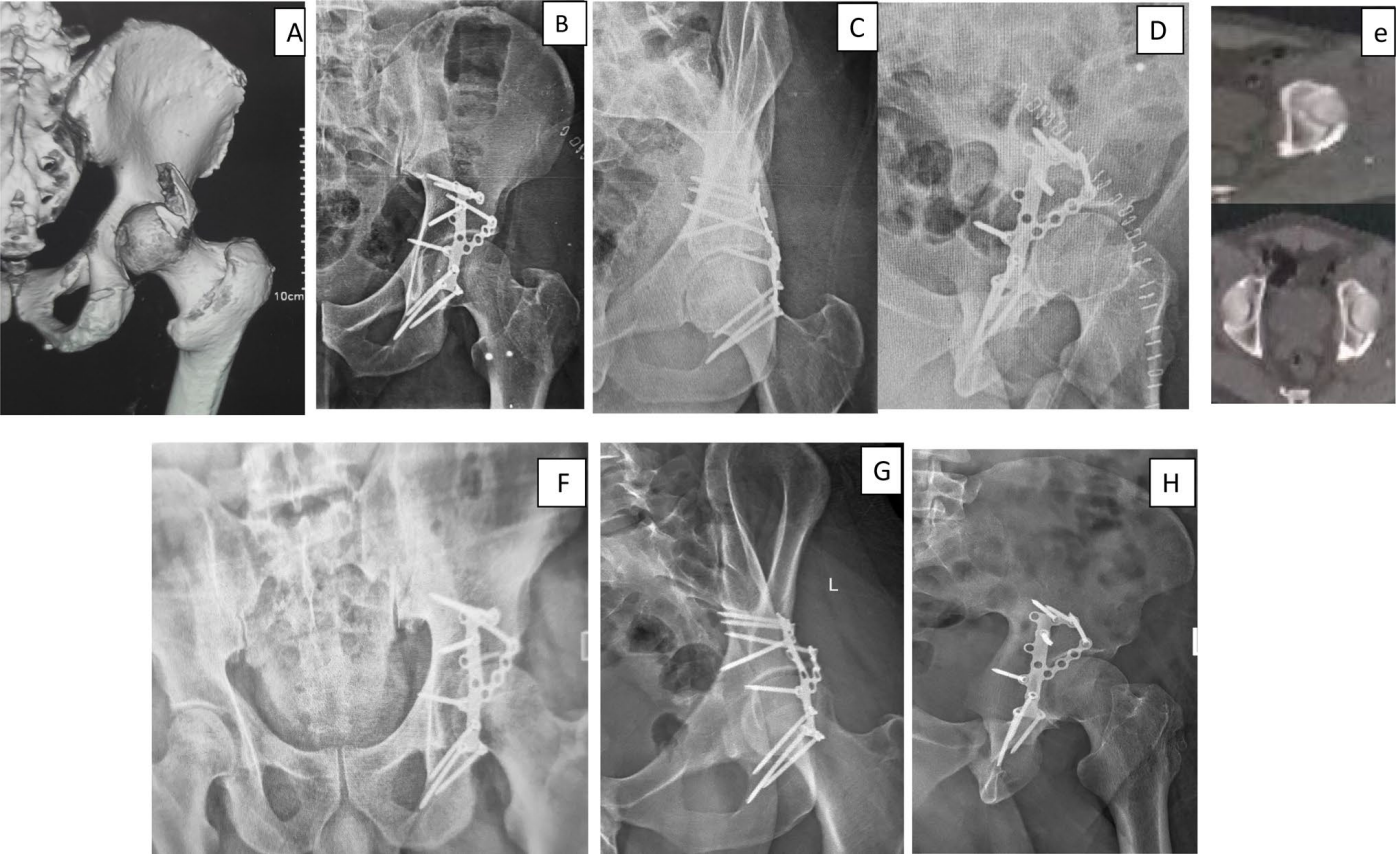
A 3D CT scan of 41 year-old male patient showing comminuted PW fracture of the left hip. B, C, D Postoperative radiograph (AP and Judet views) after calcaneal plate fixation showing anatomically reduced fracture. E Postoperative CT (axial cuts) after fixation showing anatomical reduction. F, G, H 1 year radiographs (AP and Judet views) show stable fixation with no reduction loss or fixation failure
